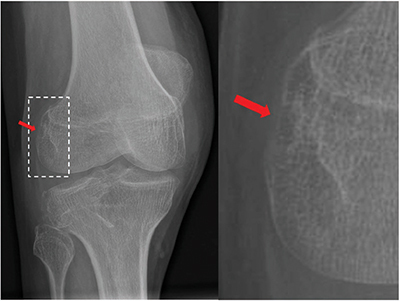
Radiography of the right knee demonstrating an erosion of the popliteal sulcus (red arrow).
